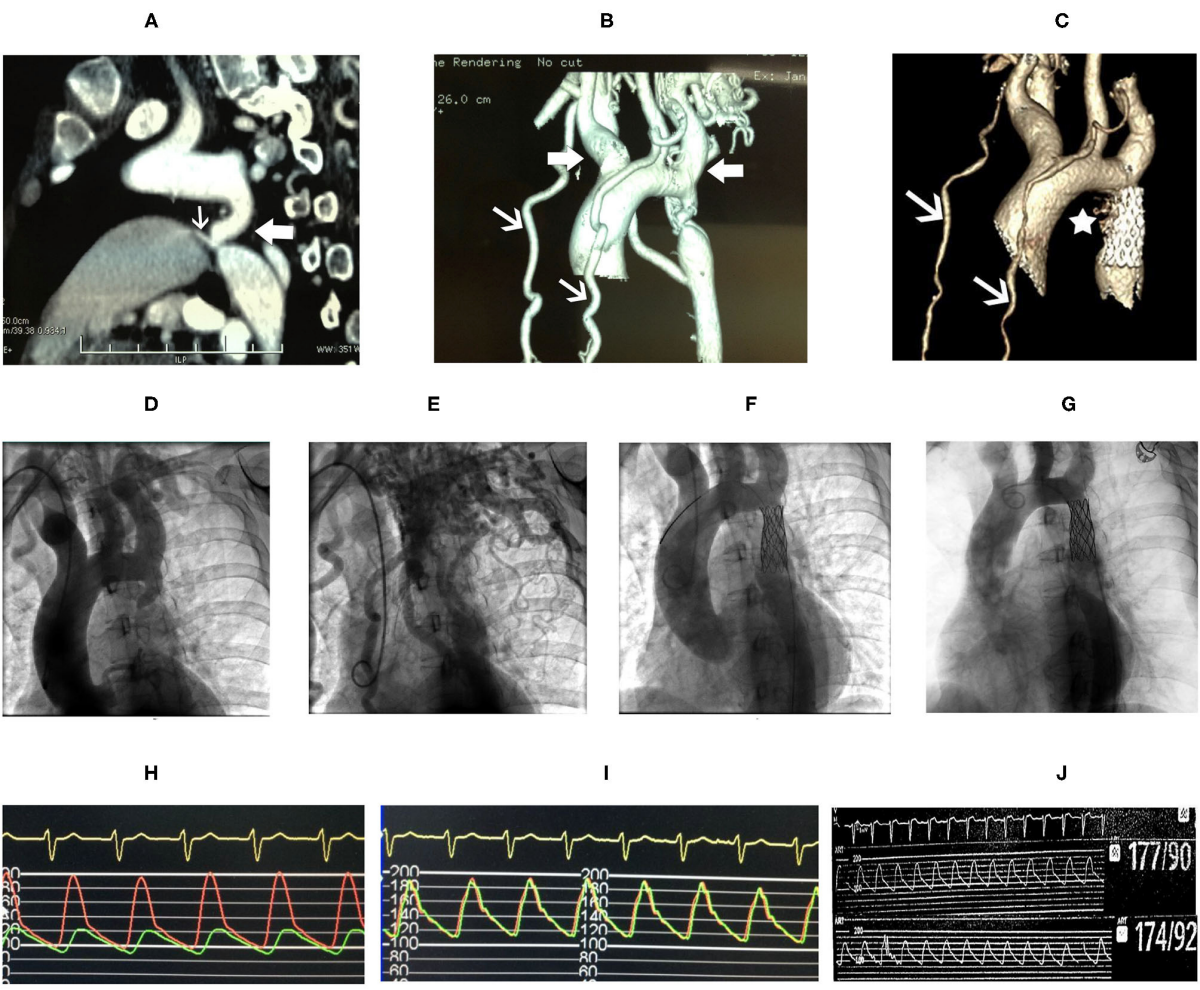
CT angiography (CTA) of the aorta (A) showed marked narrowing in the isthmic region of the descending aorta (white block arrow) and patent ductus arteriosus (PDA) (white arrow) connecting the aorta and the pulmonary artery. Dilatation of the innominate artery and the left subclavian artery (white block arrows), and extensive collateral circulation (white arrows) were shown in (B). Follow-up CTA of the aorta 8 years after stent repair showed reduced collateral circulation (C, white arrows), and the PDA is not shown (C, asterisk). Aortic angiography showed remarkable isthmic coarctation of the aorta with dilated innominate artery and left subclavian artery (D) and exuberant collateral circulation (E). Pressure gradient between the proximal and distal ends to the aortic obstruction was 74 mmHg (H). The descending aorta, after placement of stent, was shown (F) with equalization of pressure at the proximal and distal ends of the descending aorta (I). Repeated aortic angiography at 8-year follow-up showed patency of the covered stent and reduction in collateral circulation (G), and no pressure gradient was found, 177/90 mmHg at the proximal vs. 174/92 mmHg at the distal end of descending aorta, respectively (J).
